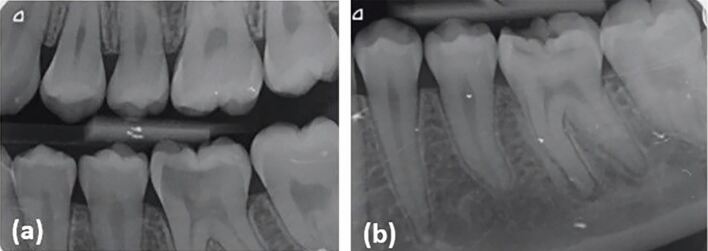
a) Pre-operative intraoral bitewing radiograph revealed extensive caries on the 36 involving the mesial pulp horn. b) Pre-operative periapical radiograph revealed evidence of apical radiolucency associated with the mesial root and periodontal ligament space widening of the distal root
